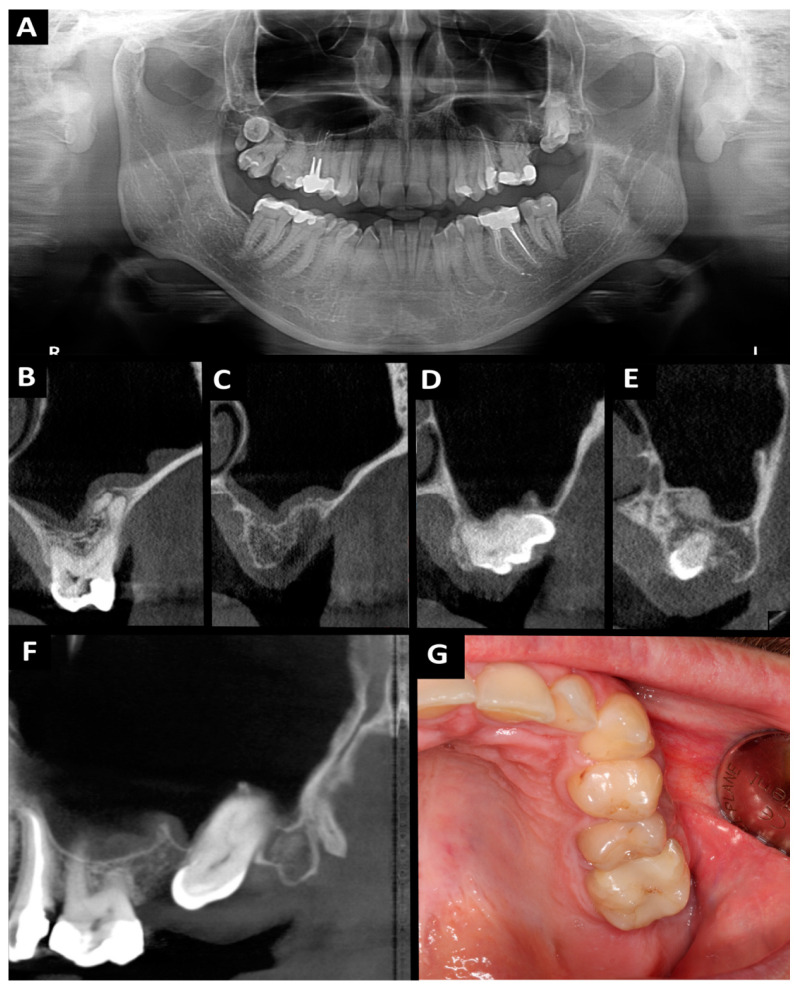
Orthopantomography (A) and CBCT (B–G) 10 months after the surgery: (A) orthopantomography; (B) cross-sectional view: tooth 26 region; (C) cross-sectional view: tooth 27 and 29′ region; (D) cross-sectional view: tooth 28 region; (E) cross-sectional view: tooth 29″ region; (F) sagittal view: tooth 26, 28 and 29″ region; (G) intraoral photograph.
